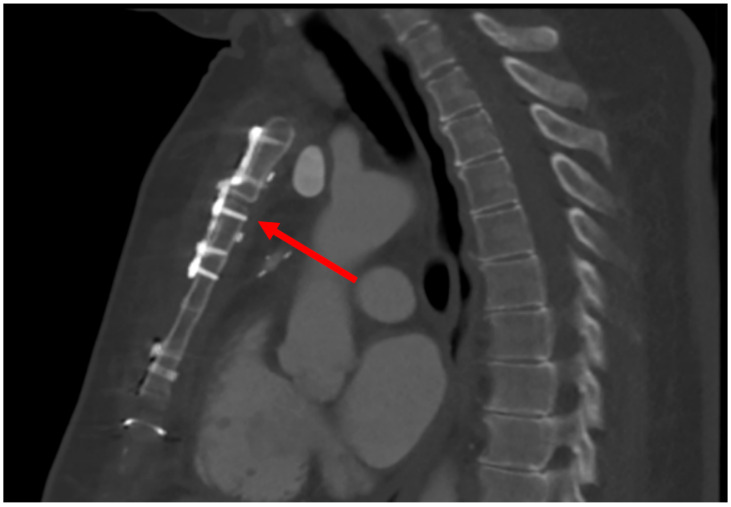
Computed tomography on postoperative day 21. The red arrow points to the tip of the screw reaching the posterior sternum lamella, demonstrating the importance of the careful measurement of screw lengths, as described in Section 2.1.
